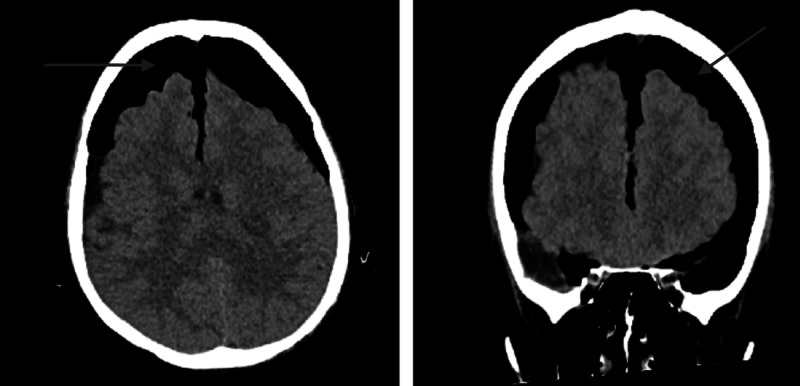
Non-contrast CT imaging in the axial (left) and coronal (right) views demonstrating the “Mount Fuji sign” for massive tension pneumocephalus. There is significant subdural air causing compression of the frontal lobes and subsequent compression away from the falx cerebri. The sulci are effaced, and ventricles have been compressed, suggestive of significant tension due to buildup of air pressure. [4]
